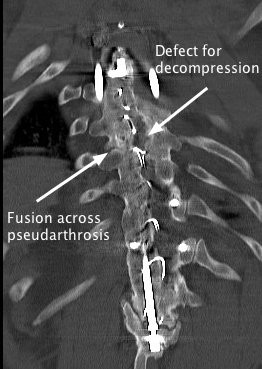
A post-operative coronal computed tomography image of the fusion across the proximal pseudarthrosis and the defect in the fusion mass through which the spinal cord was decompressed.
